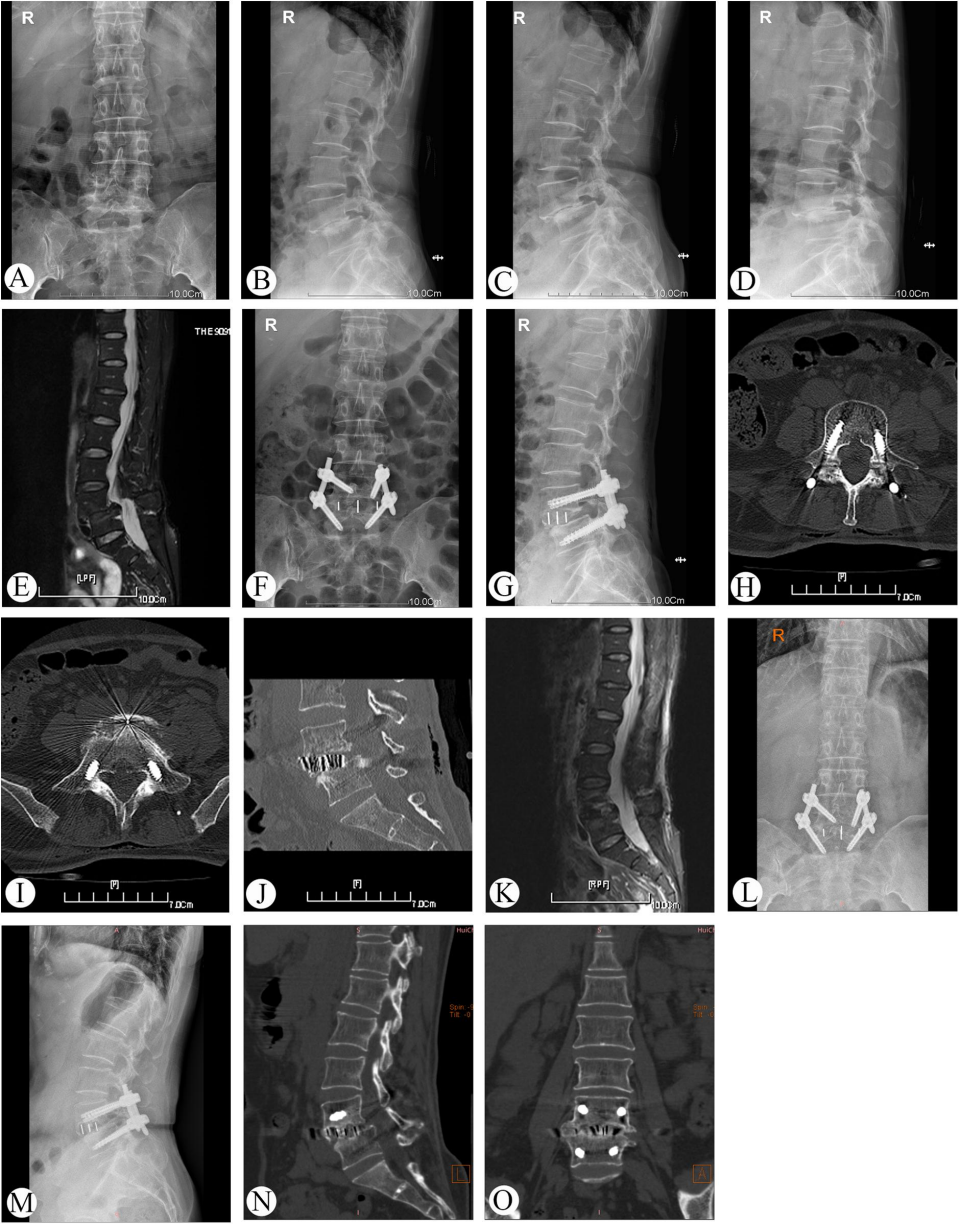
Preoperative, postoperative, and follow-up radiographs of a 56-year-old female patient who had grade II lumbar spondylolisthesis managed by single-position OLIF combined with percutaneous posterior fixation with O-arm navigation (case 13). (A–E) x-ray and MRI before operation. (F–K) x-ray, CT, and MRI at 3 days after operation. (J–O) x-ray and CT at 2 years follow-up.
